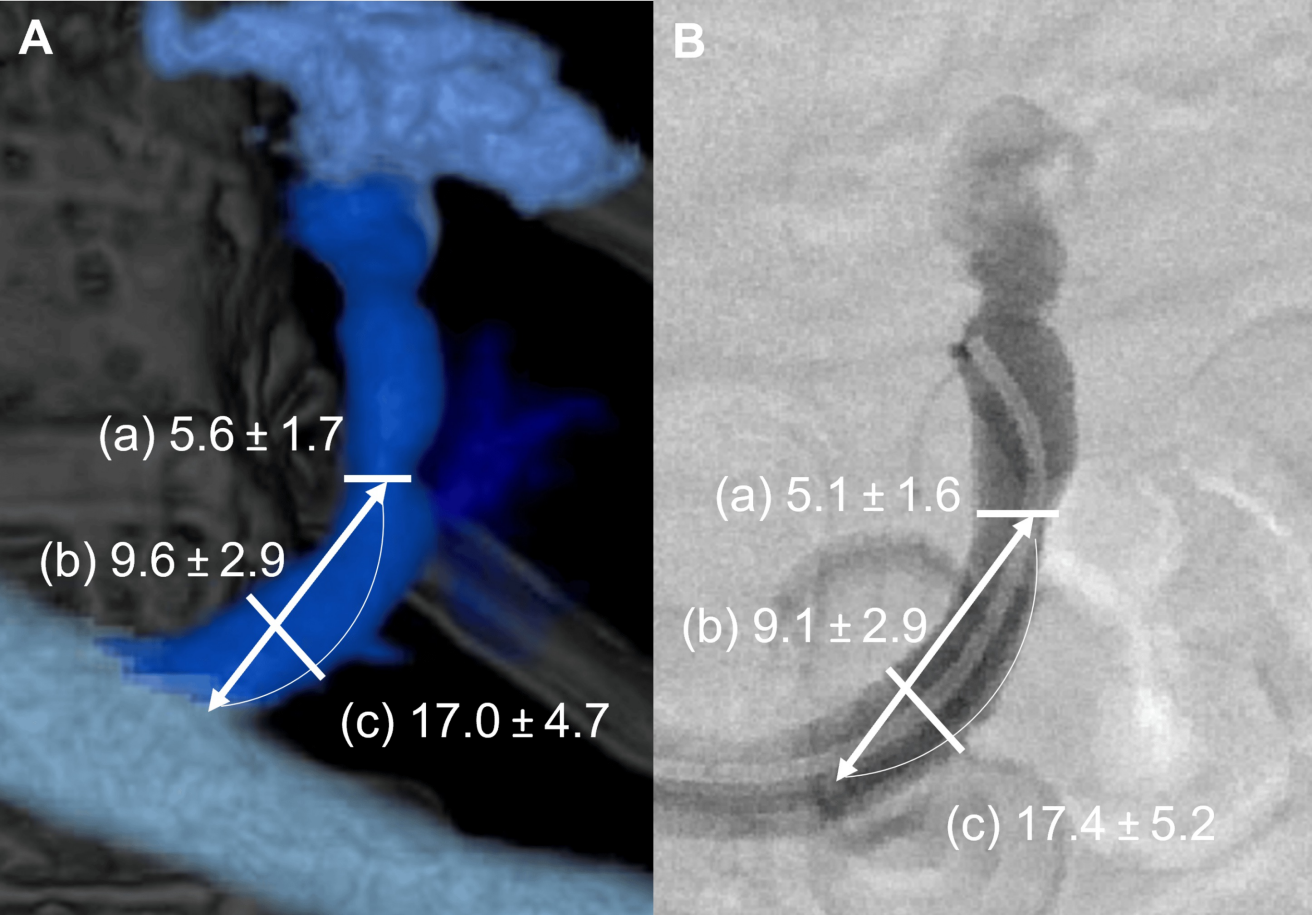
Measured values of the gastrorenal shunt outlet morphology A: 3DCT portography image with annotated measurements (in mm), including the diameter of the stenosis (a), the diameter of the common trunk (b), and the distance from the left renal vein to the stenosis (c). B: Intraoperative venography image showing the corresponding measurements of parameters a, b, and c (in mm). 3DCT: three-dimensional computed tomography
